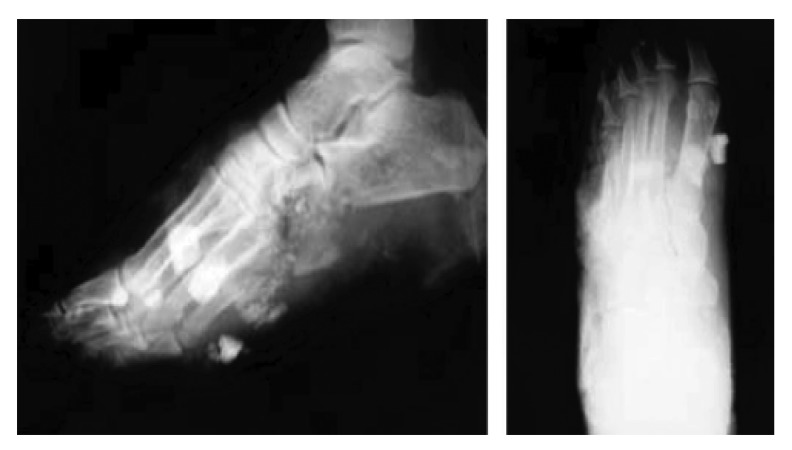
X-rays detailing multiple foreign bodies in left foot of a victim of the London bombings
